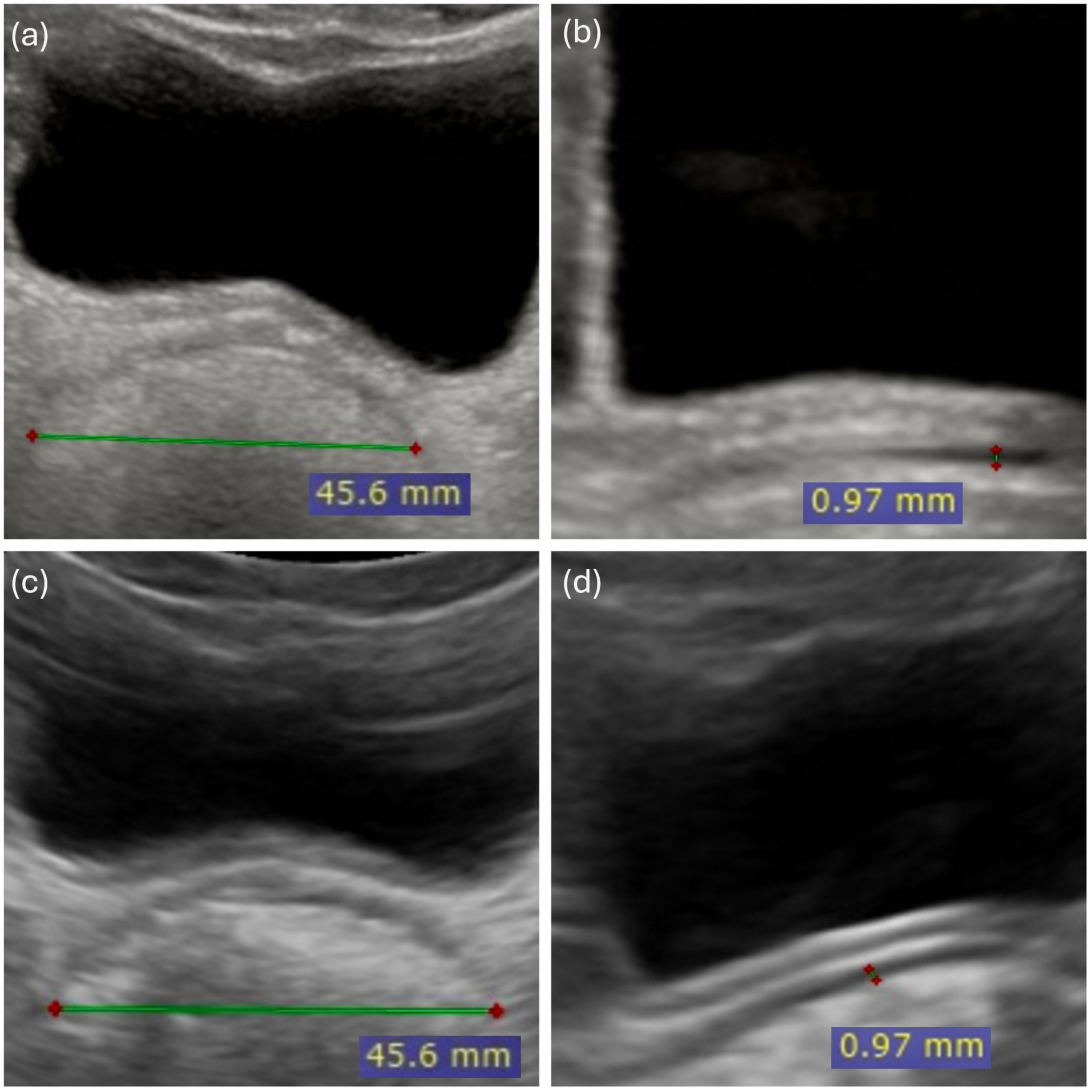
Rectal ultrasound measurements using standard and pocket-sized handheld ultra sound in a child with functional constipation. Rectal ultrasound measurements in an 8.5-year-old child with functional constipation, obtained with both standard and pocket-sized handheld ultrasound devices. Standard ultrasound: (a) Transverse rectal diameter (TRD) and (b) Rectal Anterior rectal wall thickness (RAWT). Pocket-sized handheld ultrasound (PsUS): (c) Transverse rectal diameter (TRD) and (d) Rectal anterior wall thickness (RAWT). All measurements are displayed in millimeters (mm).
